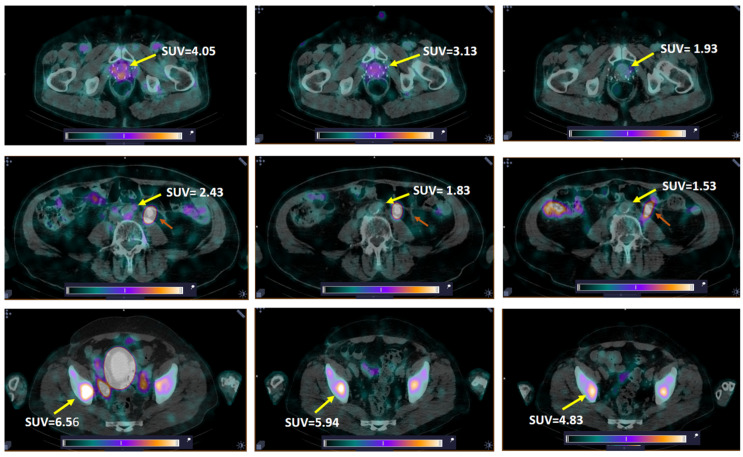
Decrease in tissue uptake (SUV_max_) in primary tumor (upper panel, Patient 7), LNM (middle panel, Patient 13, paraaortic lymph node), and BM (bottom panel, Patient 10) with time. Yellow arrow—lesion; red arrow (middle panel)—dilated left ureter. A linear relative scale (normalized at the maximum activity in the image, SUV_max,_ from 0 to 5.0) is applied.
